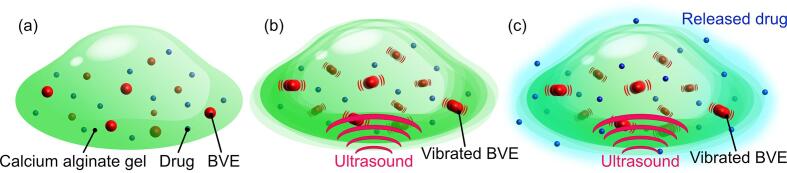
Concept of hydrogel drug release carrier having acoustic responsiveness with biocompatible bubble vibration enhancers (BVEs). (a) BVEs and drugs were encapsulated in the hydrogel carrier. (b) BVEs in the hydrogel carrier were promoted to vibrate by ultrasound. (c) Drugs were released from the hydrogel carrier because of the vibration of BVEs.
